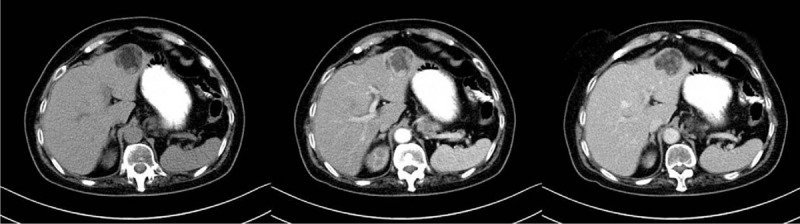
The computed tomography (CT) examination showed that in the left hepatic lobe, a kind of nearly-circular low density with a little fat solid density shadow can be seen, with a maximum diameter of 3.8 cm. After enhancement, the mass appeared to be significantly unevenly enhanced in the arterial phase and reduced in the venous phase and the delayed phase, which was considered primary hepatic carcinoma.
